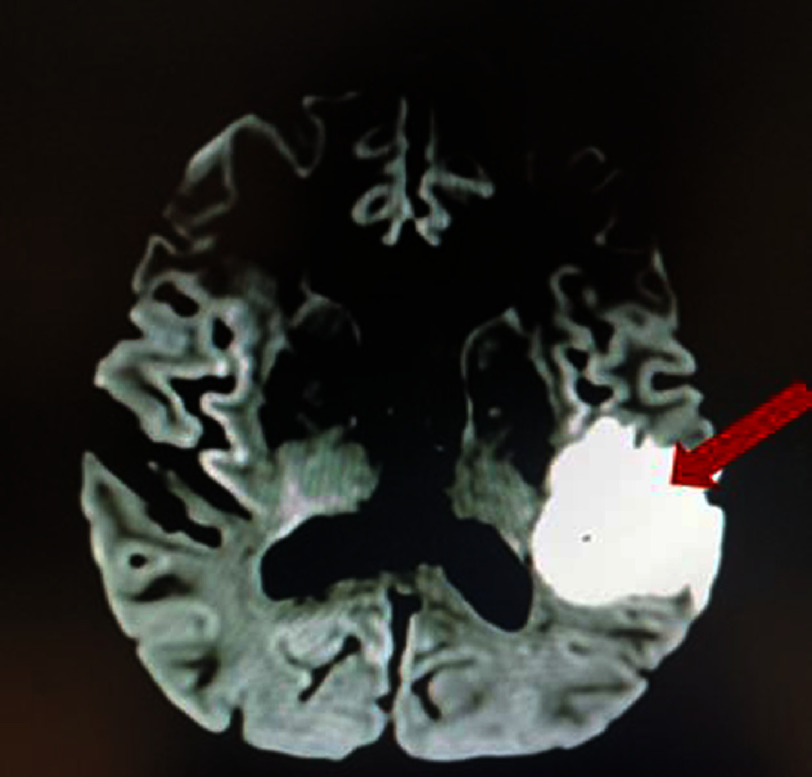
Cerebral angio-MRI showing a large stroke in the left Sylvian territory on diffusion sequence.
